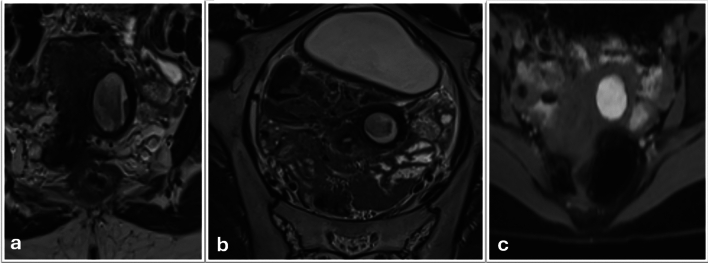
Radiological features of ACUM. a, b Coronal T2-weighted and Axial T2-weighted MRI showing left-sided myometrial mass with low T2-weighted signal rim and central intermediate to high signal. There is no communication with the endometrial cavity. c Axial T1-fat saturated image showing that the mass is of high T1-weighted signal intensity in keeping with hemorrhagic content
